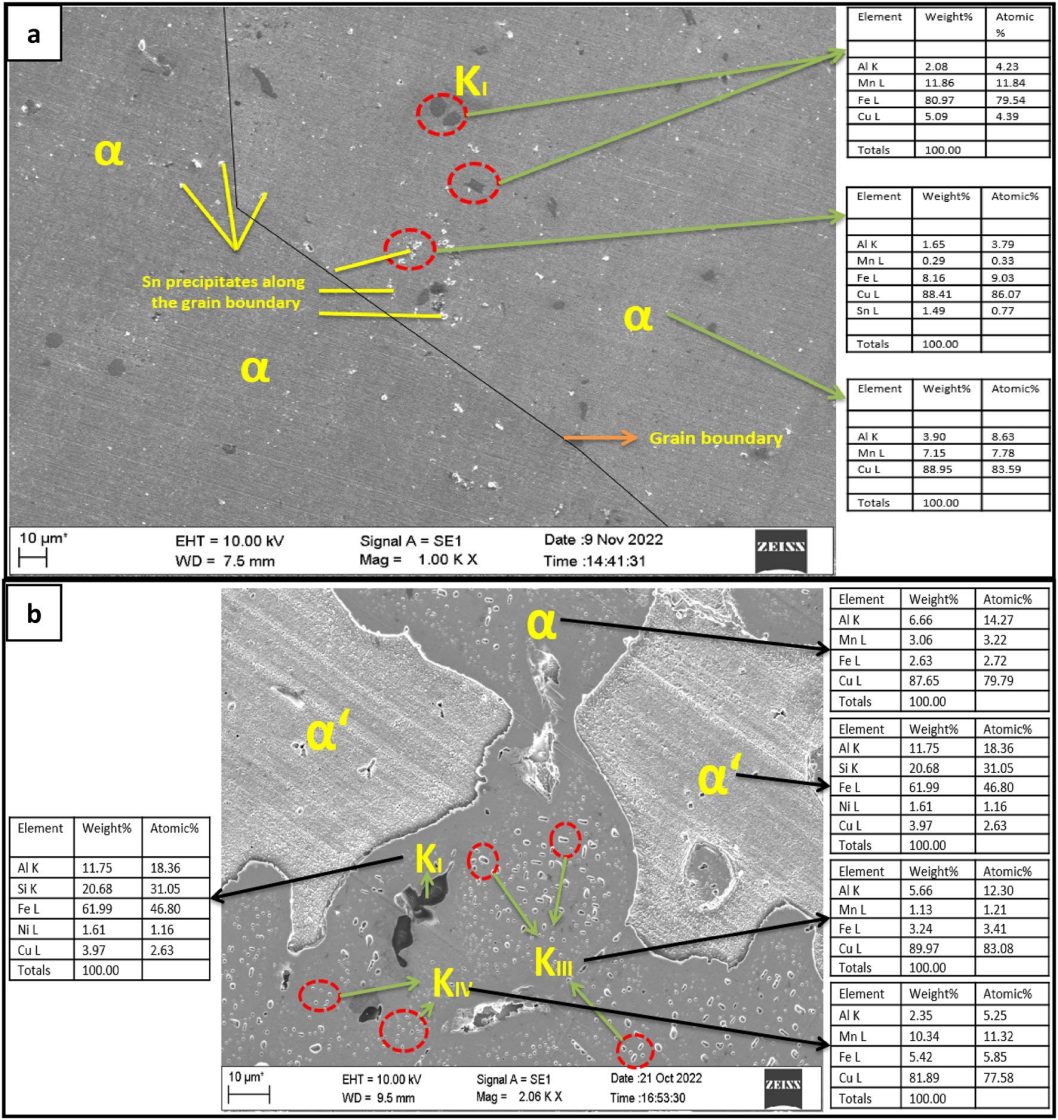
Energy dispersive X-ray metallographic image of (a) NAB with 1.5% Sn, (b) NAB with 2.5% Sn.
